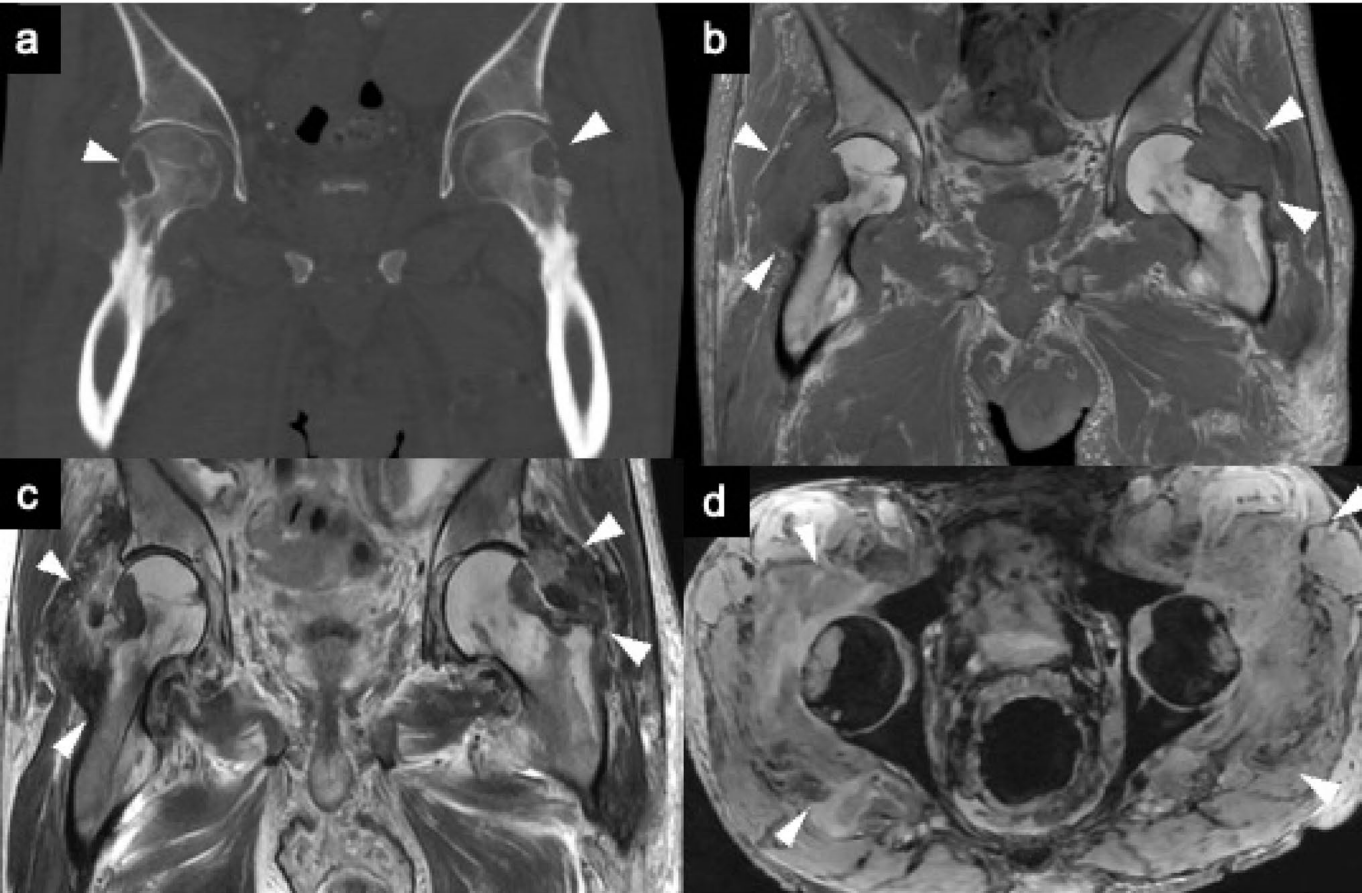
Amyloid arthropathy. Coronal T1-weighted MR image (a) shows diffuse soft tissue swelling around both hips, extending into subchondral bones (arrowheads). Coronal CT (b) reveals bone erosions with sclerotic margins (arrowheads). Axial T2-weighted (c) and T2*-weighted (d) images demonstrate intraarticular masses (arrowheads), which do not exhibit a paramagnetic effect, as evidenced by the absence of signal drop on the T2*- weighted image
